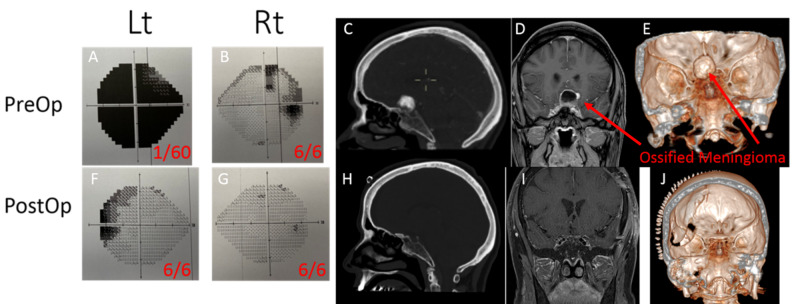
Pre and post-operative Humphrey visual field tests, acuity tests and brain imaging Figure 1: (A, B) Preoperative visual field and acuity tests. (C, D, E) Preoperative brain CT and MRI. (F, G) Postoperative visual field and acuity tests. (H, I, J) Post-operative brain CT and MRI. Abbreviations: CT, computed tomography; MRI, magnetic resonance imaging.
